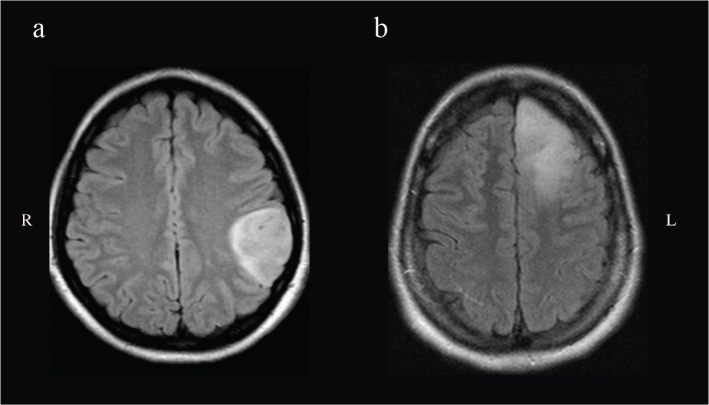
The picture shows axial FLAIR slices of two exemplary cases to demonstrate the difference in radiological tumor borders. In (a), a patient with astrocytoma harboring the left parietal lobe with well-defined tumor margins. In (b), the left frontal tumor displays irregular/diffuse margins in its posterior border.
